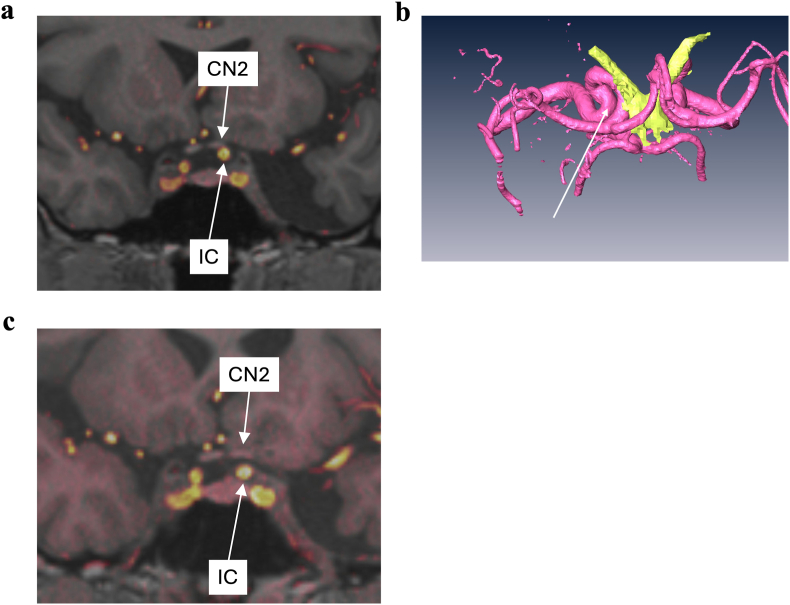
Preoperative and postoperative MRI–CT fusion images. A: Pre-operative MRI–CT fusion image showing compression of the left optic nerve (CN2) from bellow by the internal carotid artery (IC). B: 3D reconstruction confirmed The same finding. C: Postoperative MRI–CT fusion image demonstrating a clear space between the optic nerve and the internal carotid artery, indicating that the compression has been released.
